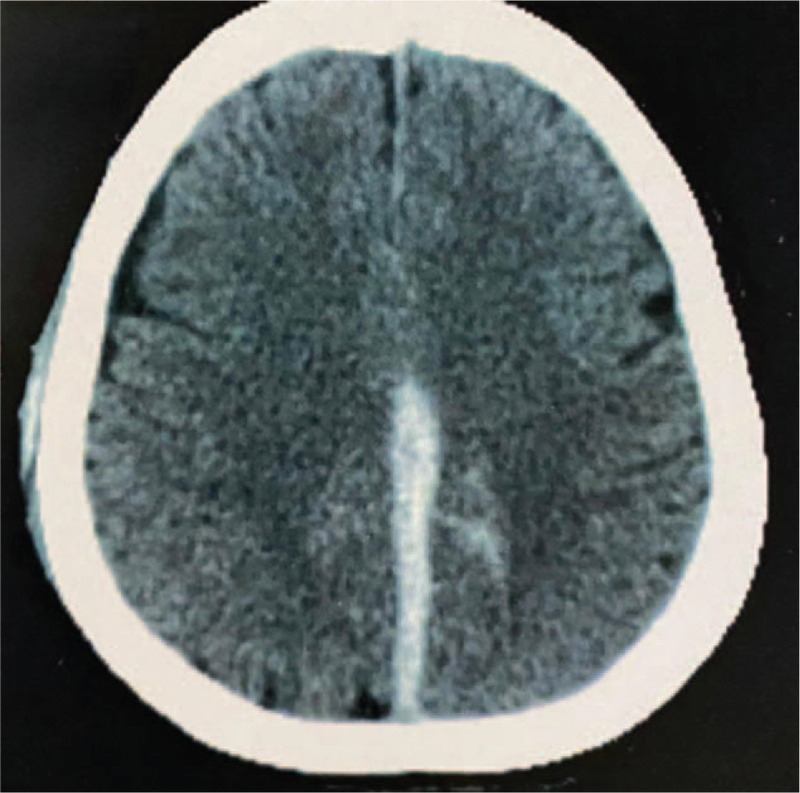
Axial CT-scans showing an acute IHSH associated with SAH (Fisher III), in a 71-year-old patient with confirmed COVID-19.
